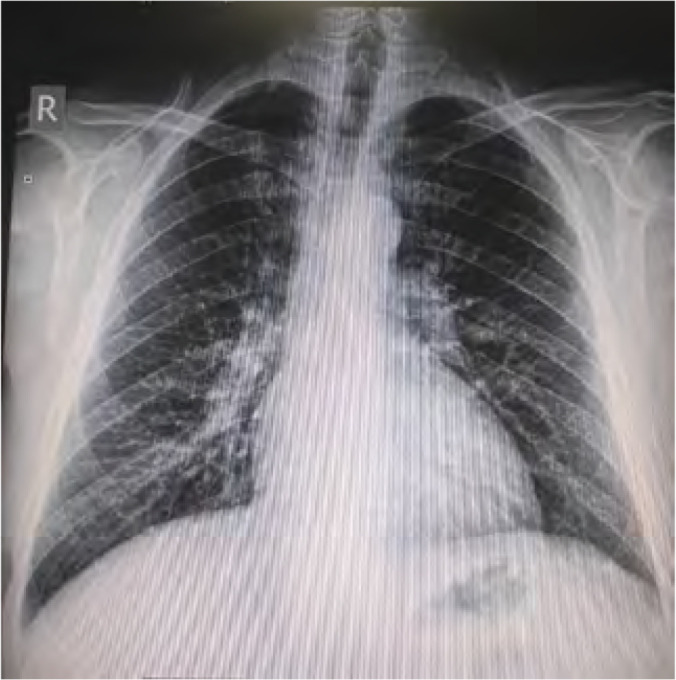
Chest x-ray did not reveal any abnormal findings.
